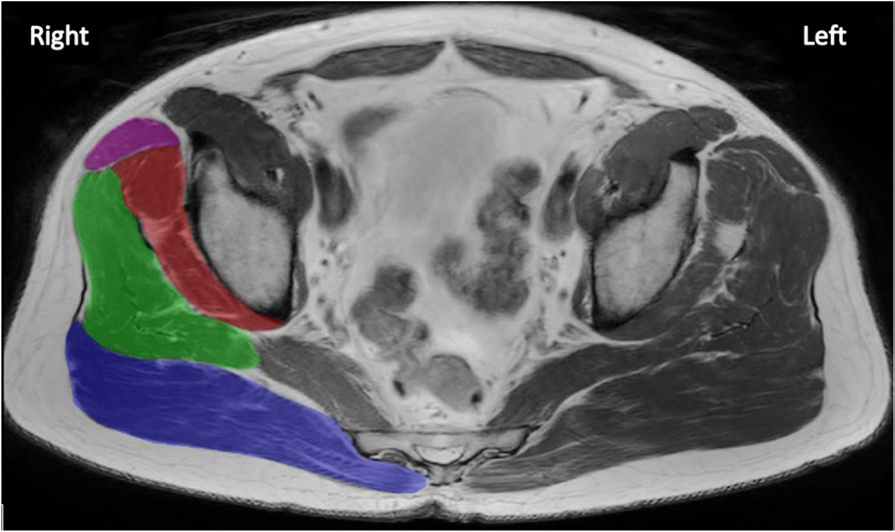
MRI tracings with manually generated masks for muscle volume calculation in an affected right hip. Note. GMax
